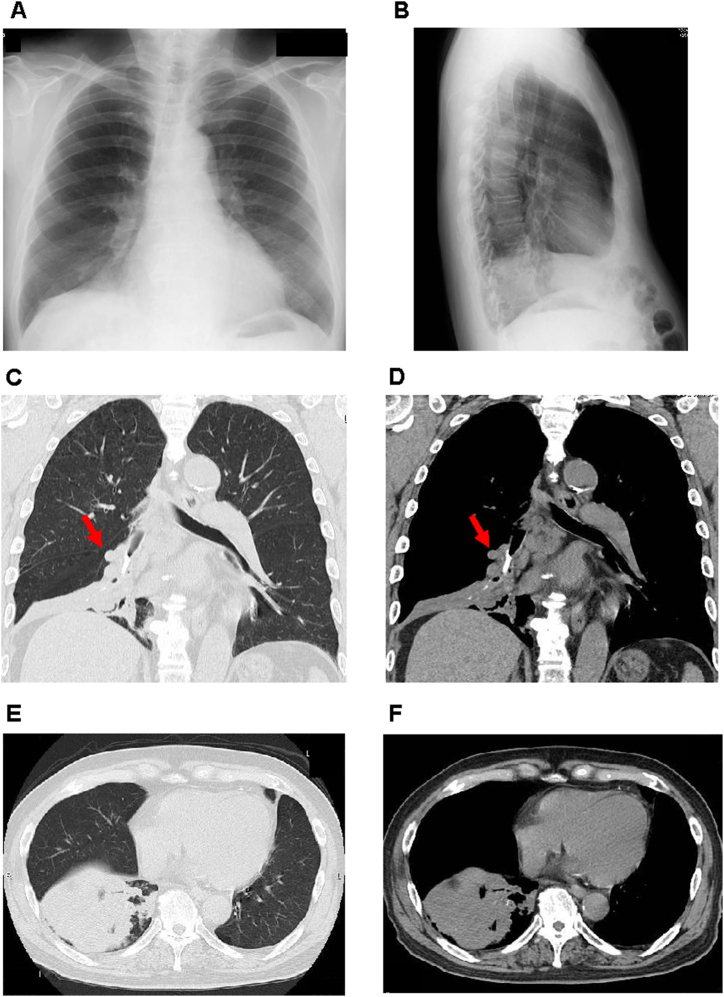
Findings in chest radiograph (A and B) and chest computed tomography (CT) (C–F). Chest radiograph showed an increase of opacity in the right lower lung. Chest CT revealed obstructive pneumonia in the right lower lung and bronchial foreign body (red arrow). (For interpretation of the references to color in this figure legend, the reader is referred to the Web version of this article.)
